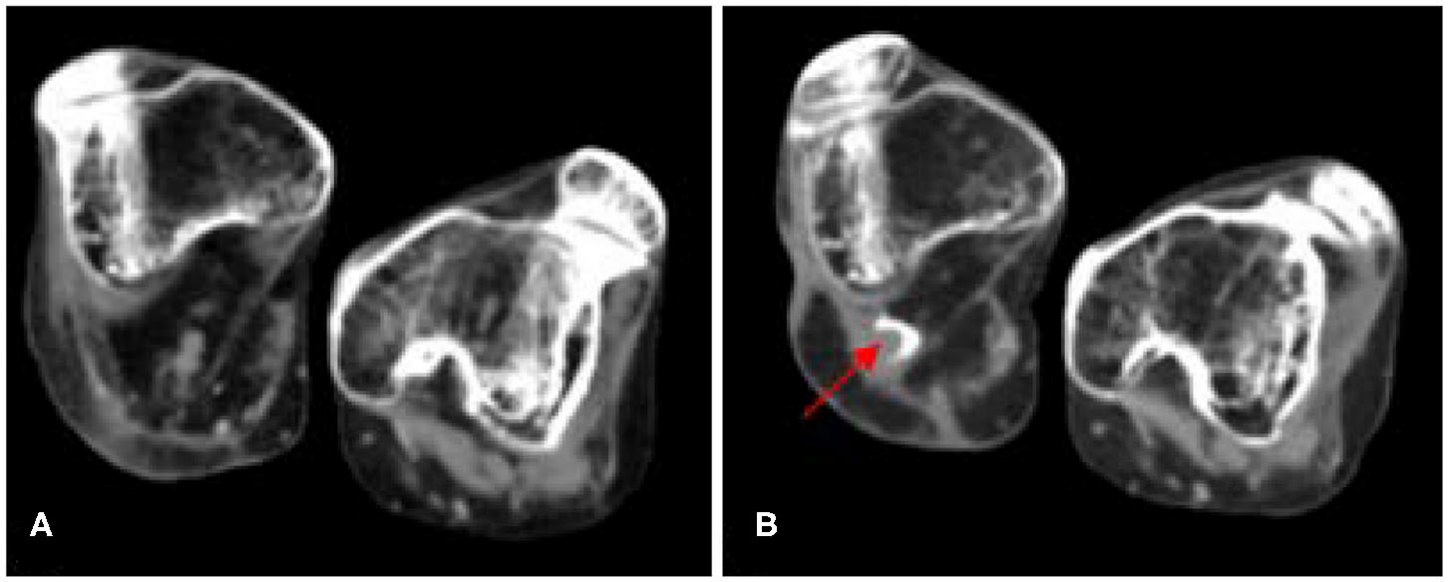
Axial Low dose CT-images at the level of the distal femur of a patient prior to and after a through-knee amputation of the right leg. (A) Eight months prior to the surgery. (B) Twelve months after the surgical procedure. Minor HO formed (4cc) on the right side posterior to the lateral femoral condyle (red arrow). FOP, fibrodysplasia ossificans progressiva; HO, heterotopic ossification; CT, computed tomography.
